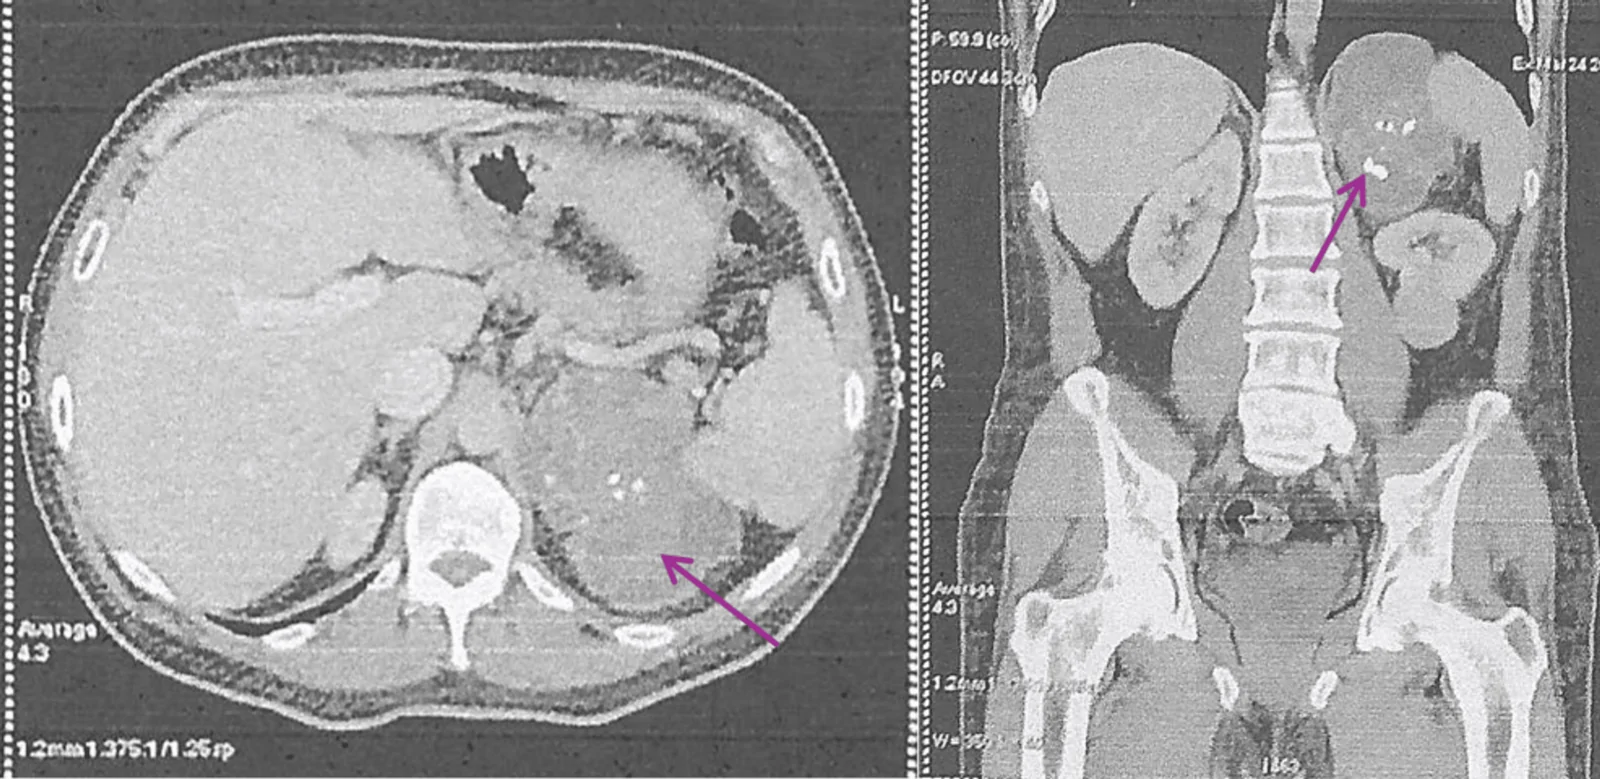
Computed tomography (CT) images (axial and coronal views) demonstrating an adenomatoid tumor of the adrenal gland
